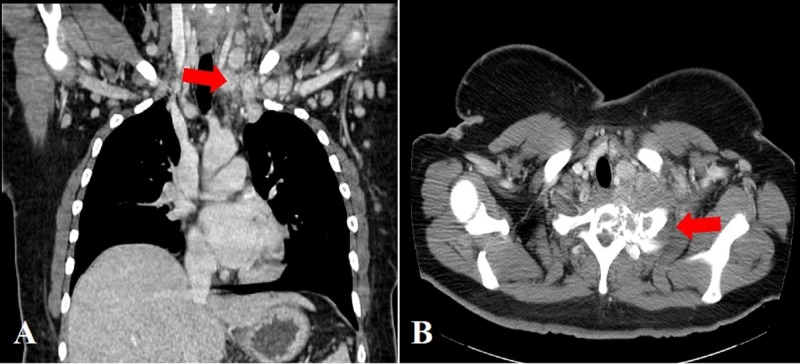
Computed tomography (CT) scan showing A) cervical lymphadenopathy B) 6 x 4.5 cm mass in the para-spinal muscle of the thoracic inlet invading the T1 and posterior part of the first rib with a pathologic compression fracture
